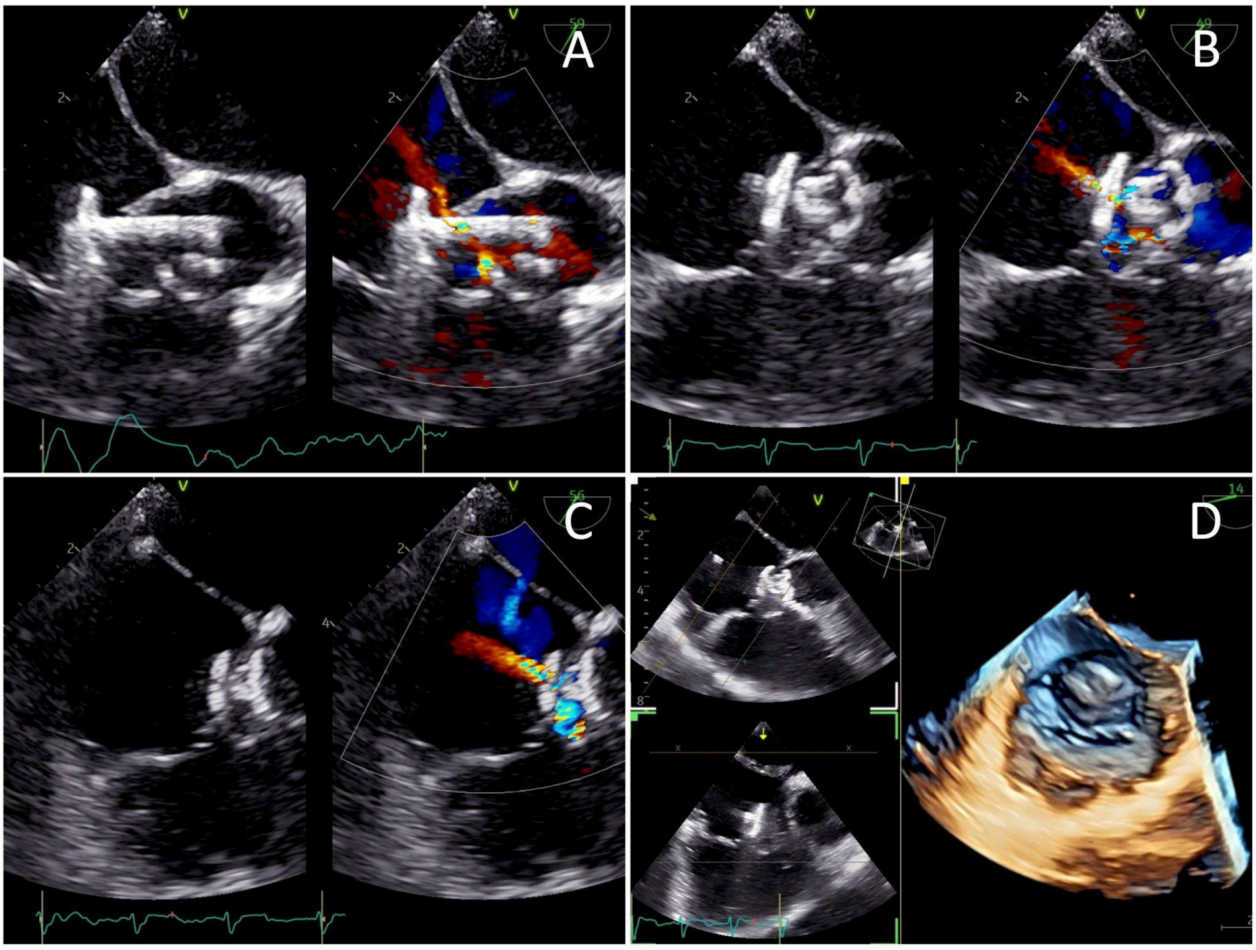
Transesophageal echocardiography views of (A) the low-pressured disc being deployed at the right atrium before the whole system was pulled into the tricuspid valve defect, (B) the high-pressured disc deployed at the left ventricle ends to seal the defect, and (C) a minimal residual central shunt with no peripheral leakage. (D) Post-procedure images demonstrating the device successfully sealing the defect.
